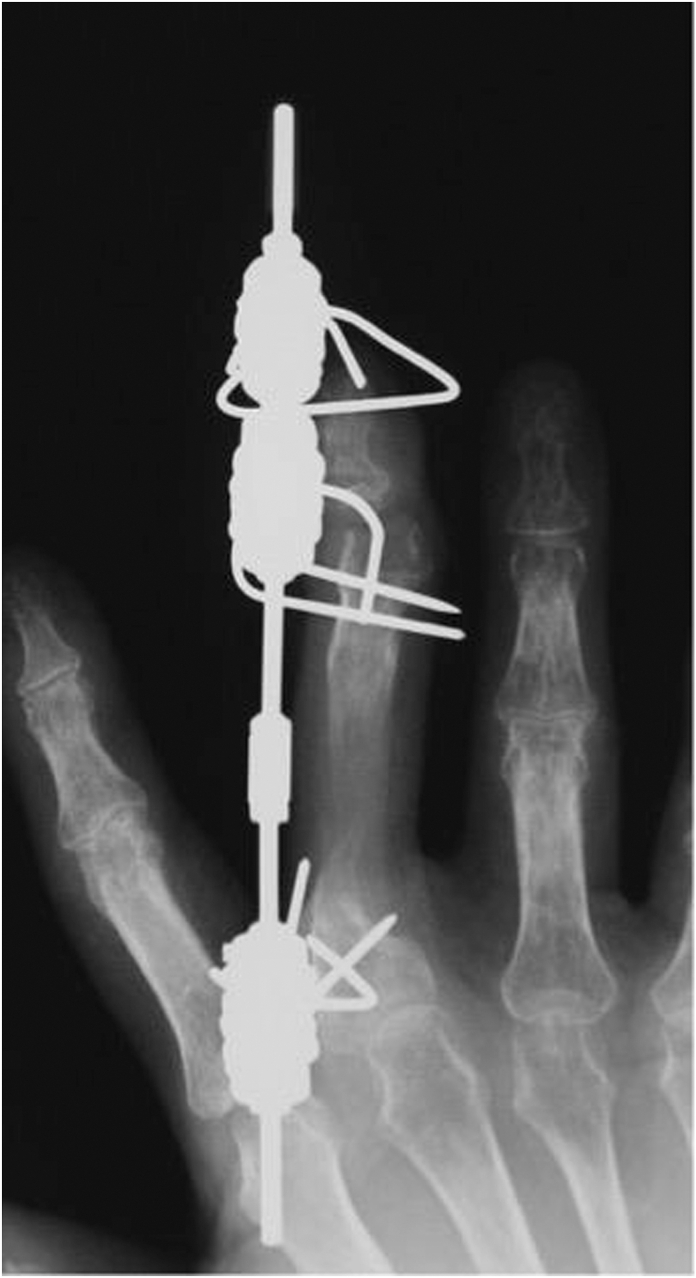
X-ray shows the callus formation after gradual lengthening of the proximal phalanx before callus flexion.
